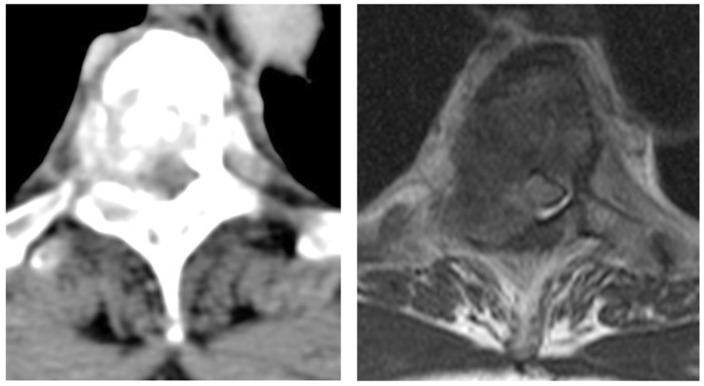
Example of high-grade (Bilsky grade 3) MESCC in a 43-year-old woman with breast cancer. Axial CT was performed in the portal-venous phase (left) with matching axial T2-weighted MRI (right) [43].
